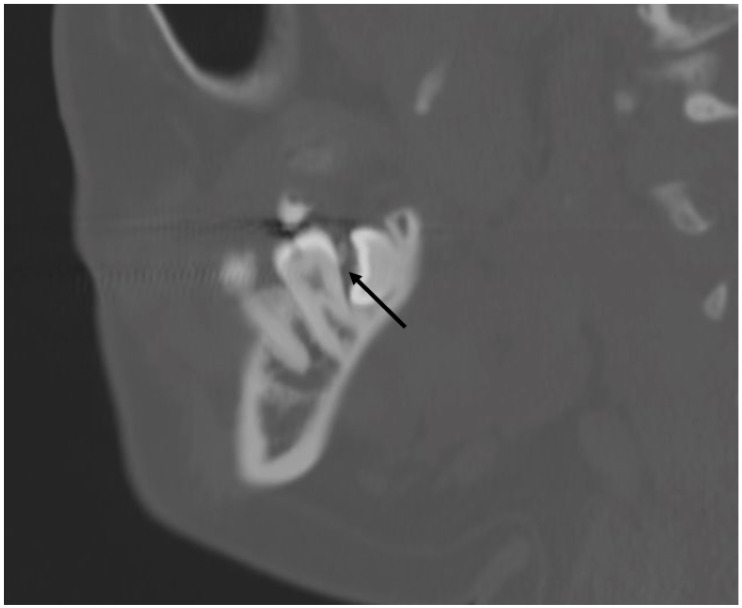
Sagittal section CT showing a vertical bony defect (arrow) posterior to the mandibular second molar, indicative of periodontal disease. In this case, the mesial-angulated third molar is contributing to the vertical bony defect, precluding adequate hygiene.
